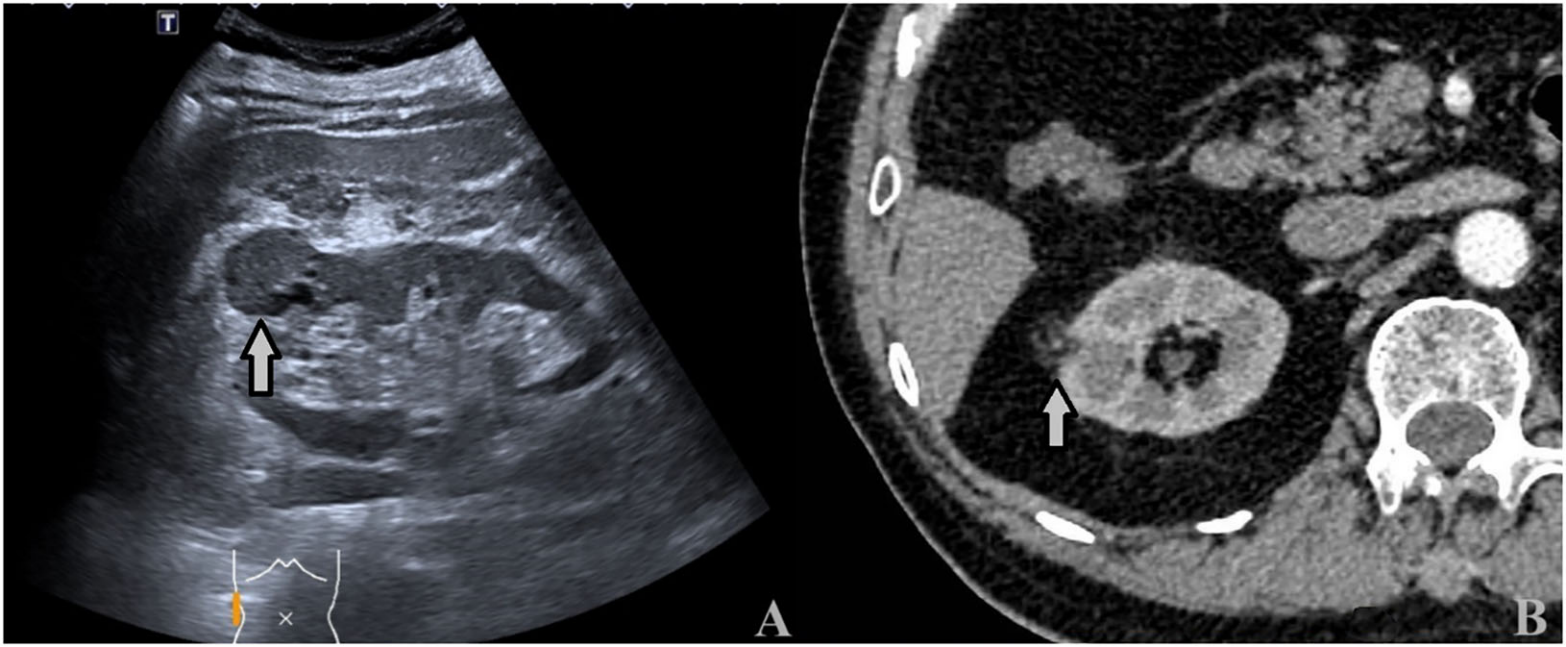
A 64-year-old man with a 3.2 × 2.8cm ccRCC. (A) Ultrasound showed a heterogeneous hypoechoic mass with intratumoral fluid areas located at the upper pole of the right kidney (arrows). (B) CECT: the tumor showed inhomogeneous hyperenhancement with many unenhanced areas (arrows).
